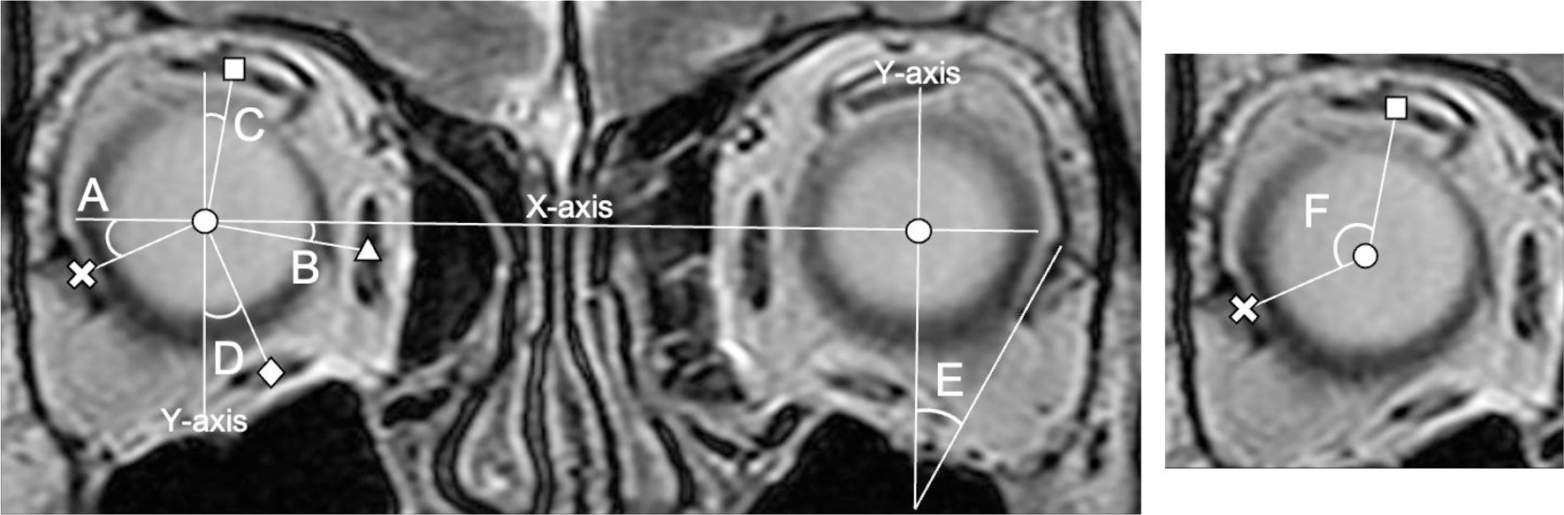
Details of the extraocular muscle malpositioning evaluation by standard coronal magnetic resonance imaging. Vertical angle of the horizontal muscle (A, B), horizontal angle of the vertical muscle (C, D), tilting angle of the lateral rectus (LR) muscle (E), and superior rectus (SR)–LR displacement angle (F). Circle (●), globe center; cross (×), LR center; triangle (▲), medial rectus center; square (■), SR center; diamond (◆), inferior rectus center.
